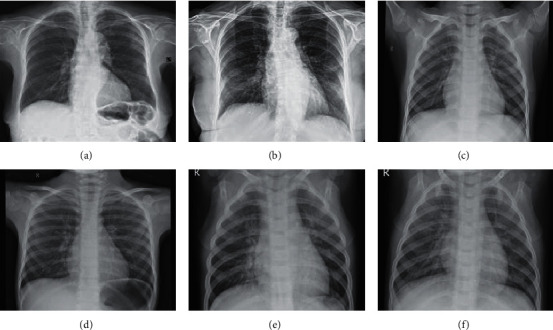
Cases of chest X-ray images: (a-b) COVID-19 images; (c-d) normal images; (e-f) other pneumonia images.
